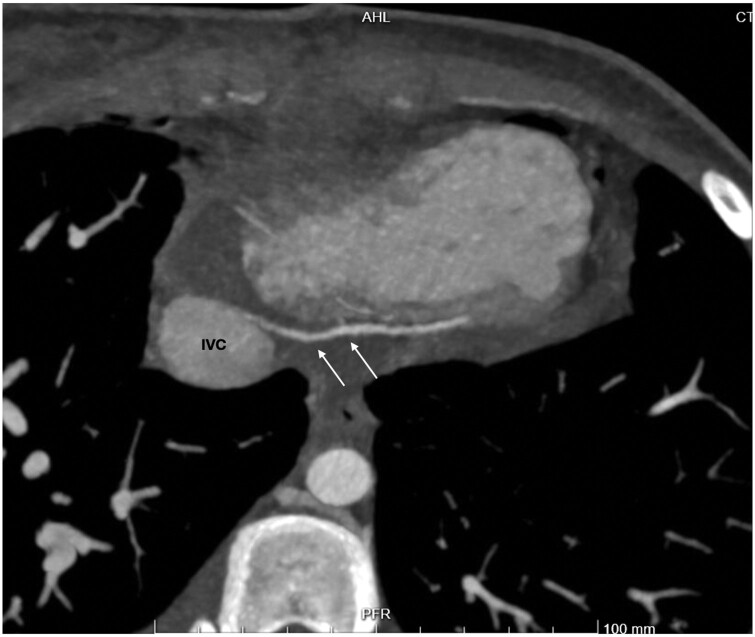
Computed tomography image demonstrating the anomalous small cardiac vein (arrows). IVC: inferior vena cava.
